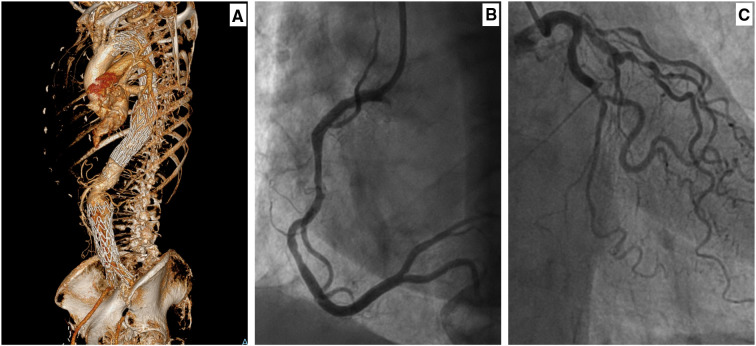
A female in her seventies with a saccular aneurysm in the descending thoracic aorta (10). She had thoracic endovascular aortic repair (TEVAR) in 2015 and subsequently underwent endovascular repair of her infrarenal aortic aneurysm in 2018. Her background history included ex-smoker, hypertension, lipid disorder, and right femoral-popliteal percutaneous transluminal angioplasty. (A) A 3D-CTA reconstruction, showing thoracic endograft. Following the TEVAR, the patient complained of intermittent chest pain and shortness of breath. A coronary angiogram was performed after her symptoms worsened. (B) Coronary angiogram (right main coronary artery) with no evidence of occlusive coronary disease. (C) Coronary angiogram (left main coronary artery) with no evidence of occlusive coronary disease.
